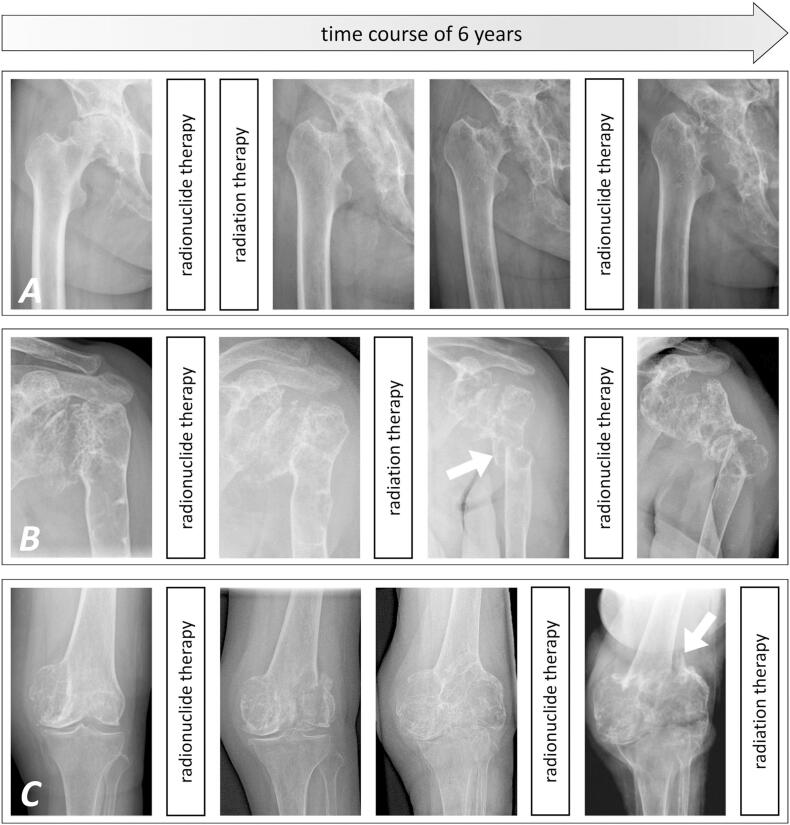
Anteroposterior X-rays of the right hip (A), the left shoulder (B) and the left knee joint (C) from similar time points demonstrating the rapidly progressive osteolytic destruction of the respective long bone segments and diarthrodial joints over the course of six years. These three locations have been selectively treated by radiation therapy (at the left knee following endoprosthetic replacement) while symptomatic off-label systemic radionuclide therapy by 153Sm-EDTMP was administered repeatedly and improved pain at all anatomic locations. White arrowheads in B and C point at the sites of pathological fractures of the left proximal humerus and distal femur diaphysis.
